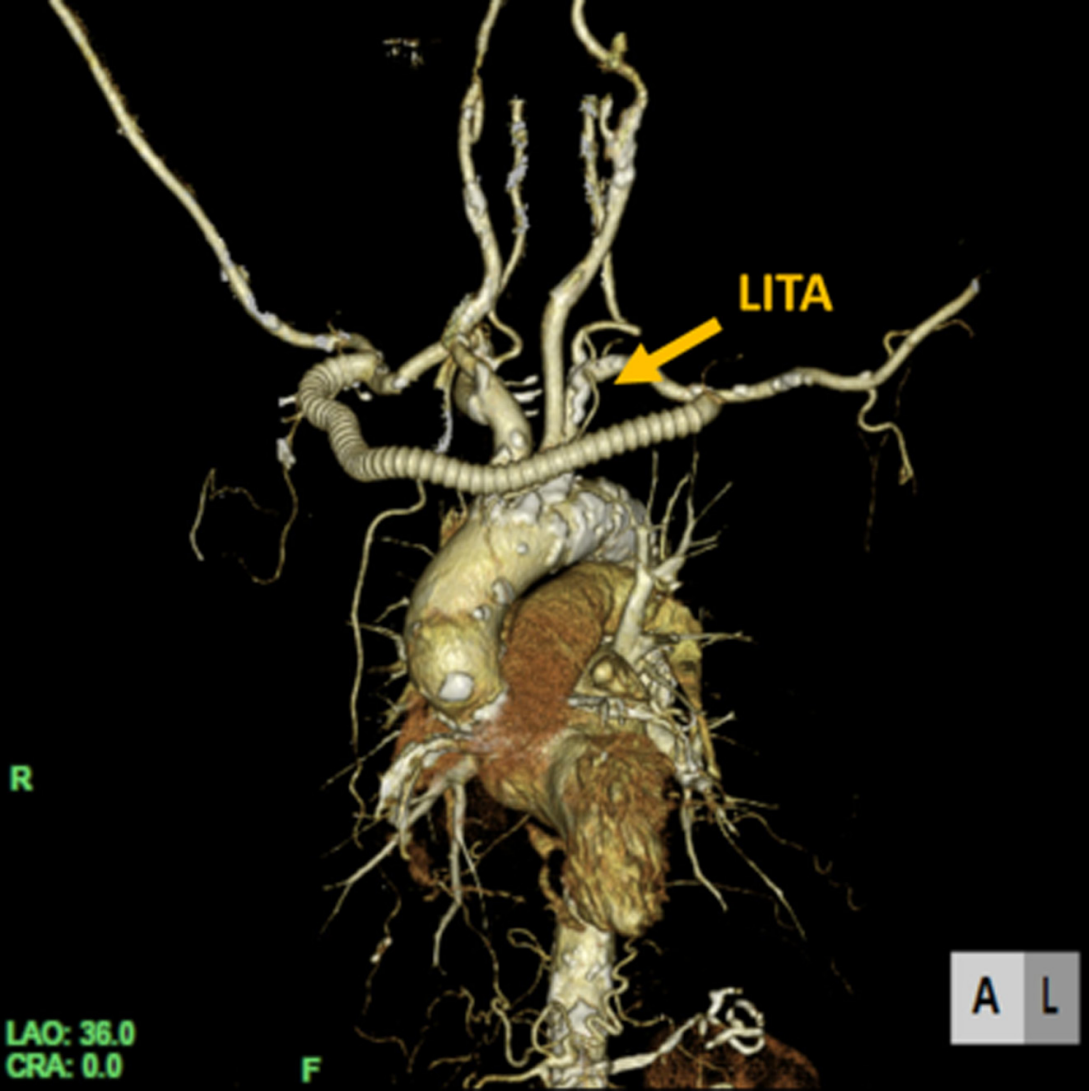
Postoperative contrast‐enhanced computed tomography revealed that the artificial blood vessel patented and anastomosis site had no abnormalities.
